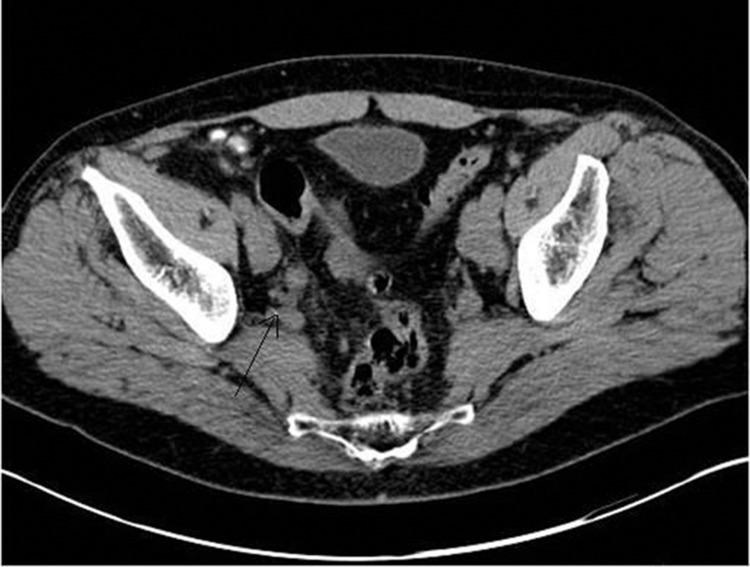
Computed tomography showing right ureteric thickening and local lymphadenopathy
